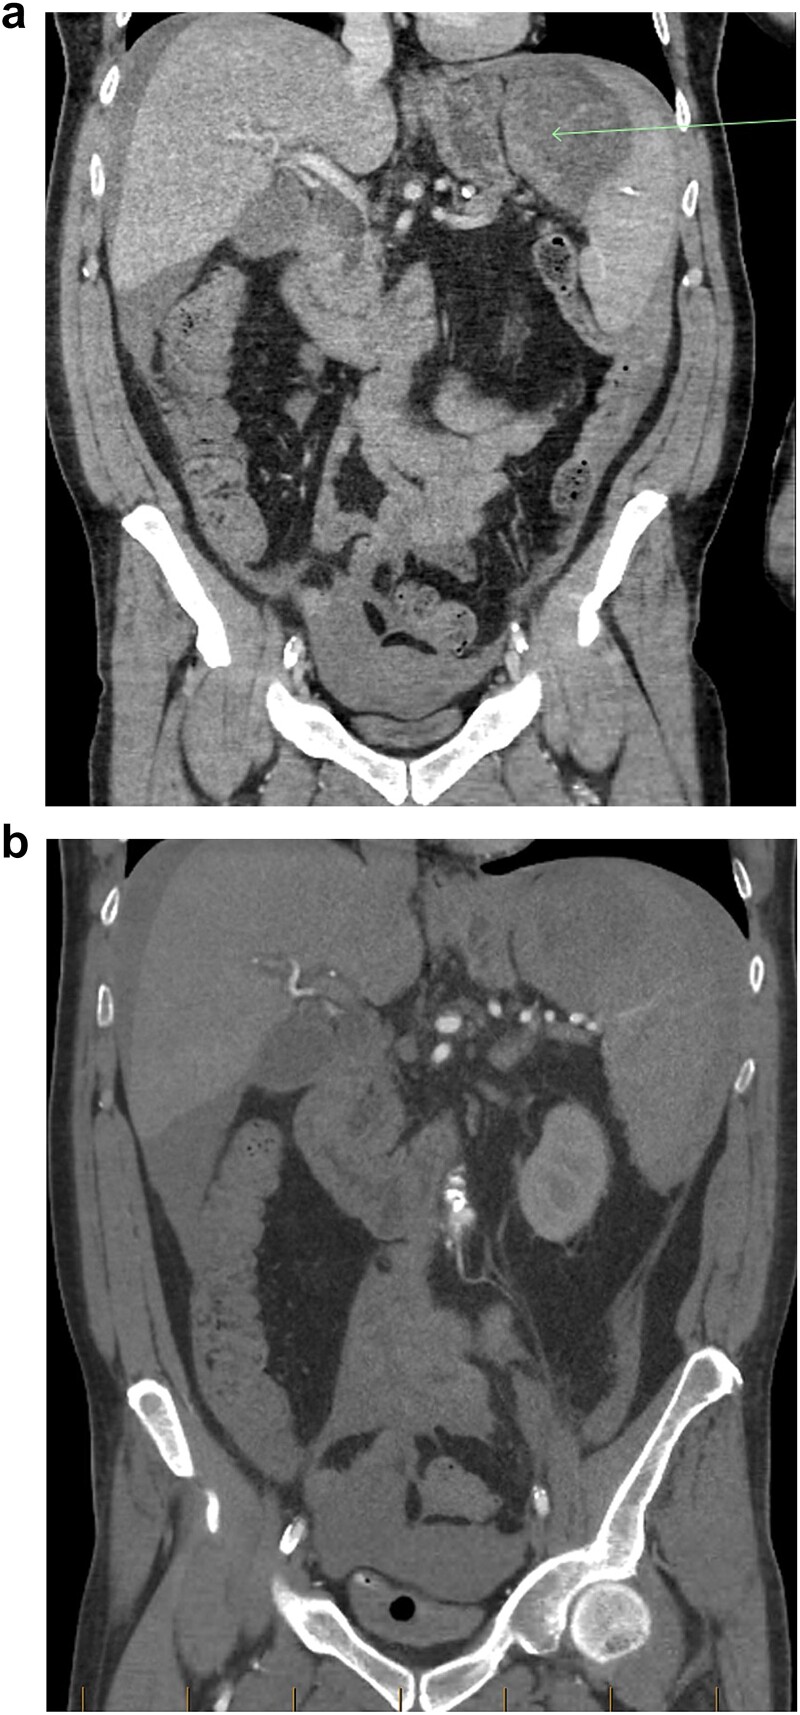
(a) Coronal view of CT-abdomen/pelvis in the portal venous phase showing moderate volume free-fluid/blood around the liver, both paracolic gutters, extending to the lower abdomen and pelvis. There is a large haematoma seen within or adjacent to the spleen (arrow). (b) Coronal view of CT-abdomen/pelvis angiogram redemonstrating the known splenic haematoma, however there was no evidence of active arterial contrast extravasation.
